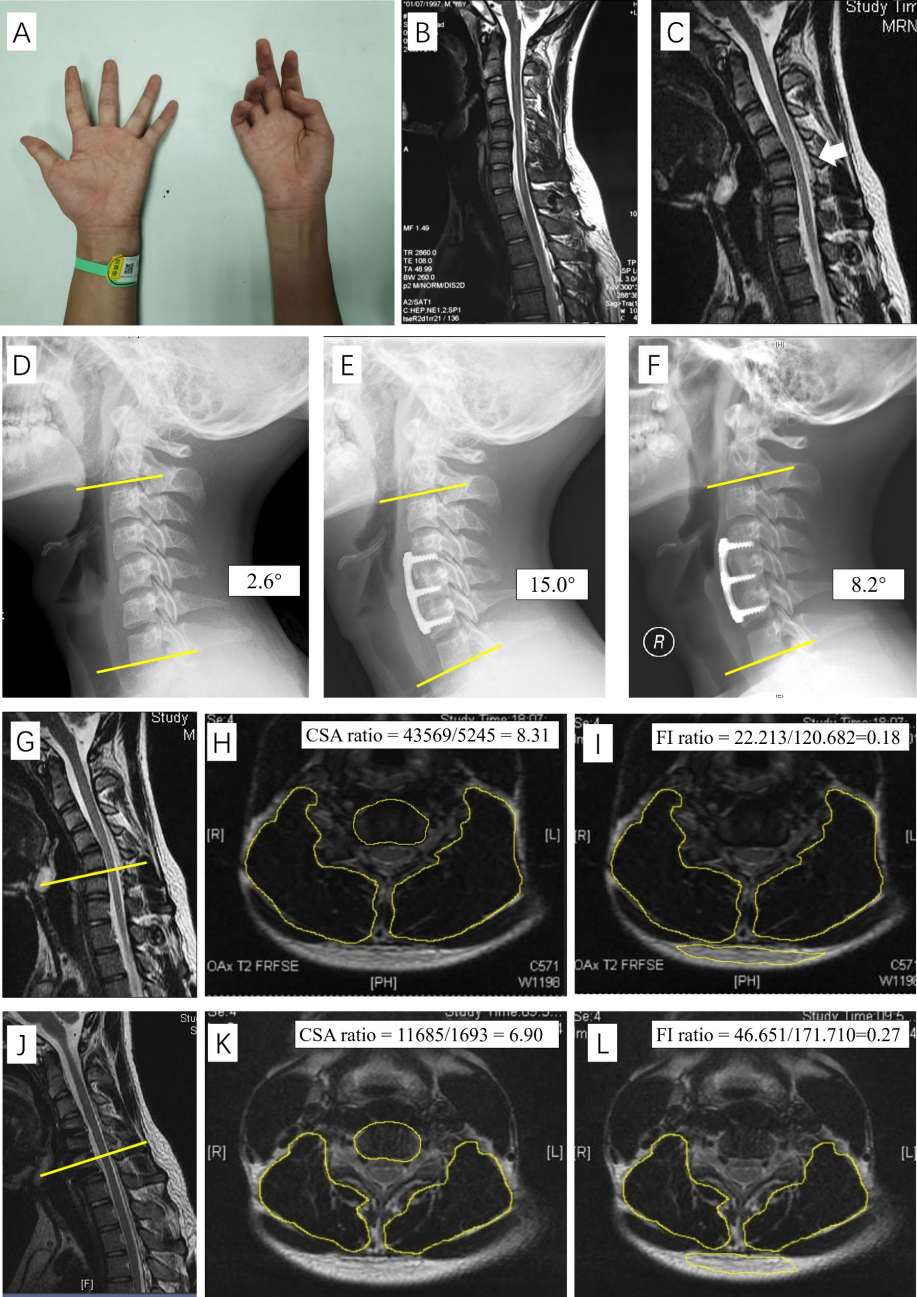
Preoperative and postoperative imaging assessments in a patient with known Hirayama disease. A: Significant amyotrophy of the intrinsic hand on the right side. B: In the neck-neutral position, no significant abnormality on cervical MRI is observed. C: In the neck-flexion position, forwards-shifting of the posterior dura and a crescent-shaped LOA behind the posterior dura on MRI are shown (white arrow). D: Preoperative assessments of cervical sagittal alignment (C2-7 Cobb method). E: Postoperative immediate assessments of cervical sagittal alignment (C2-7 Cobb method). F: Postoperative 2-year assessments of cervical sagittal alignment (C2-7 Cobb method). G: Preoperative localization of the C5-6 segment on neck-flexion MRI. H: Preoperative measurements of the cross-sectional area of the posterior cervical muscles. I: Preoperative measurements of the fatty infiltration of the posterior cervical muscles. J: Postoperative localization of the C5-6 segment on neck-flexion MRI. K: Postoperative measurements of the cross-sectional area of the posterior cervical muscles. L: Postoperative measurements of the fatty infiltration of the posterior cervical muscles. MRI: Magnetic resonance imaging. LOA: Loss of dorsal dural attachment from the pedicle
